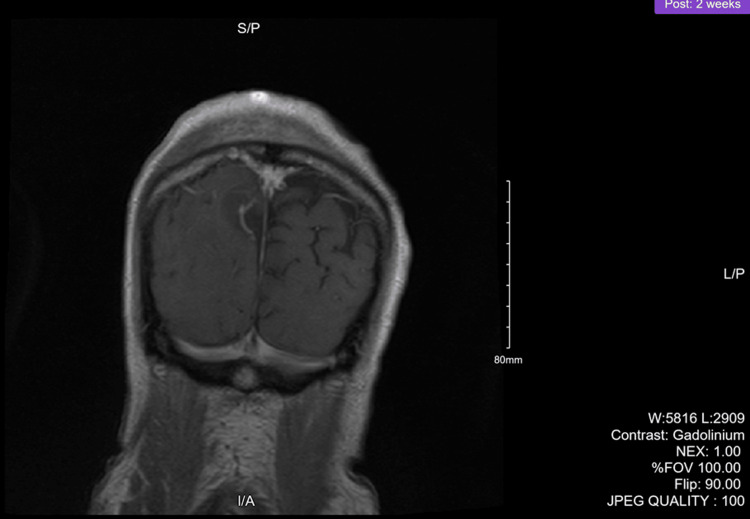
Postoperative brain MRI sagittal view MRI: magnetic resonance imaging
